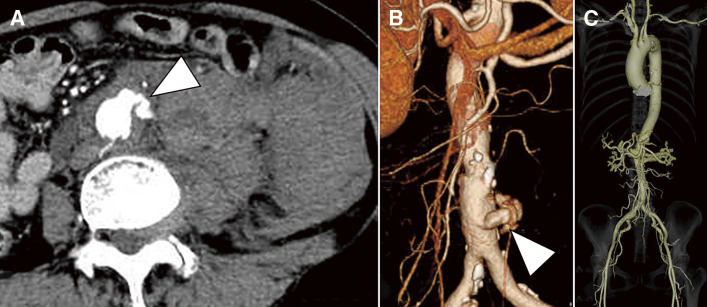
Fig. 1 Preoperative CT findings. Preoperative contrast-enhanced CT reveals extravasation of the contrast from the infrarenal abdominal aorta (arrowhead), accompanied by a massive retroperitoneal hematoma (A). No apparent aneurysmal changes, dilatation, or stenosis were observed in the entire aorta, including the thoracic aorta, abdominal aorta, and their branches (B, C). CT: computed tomography
